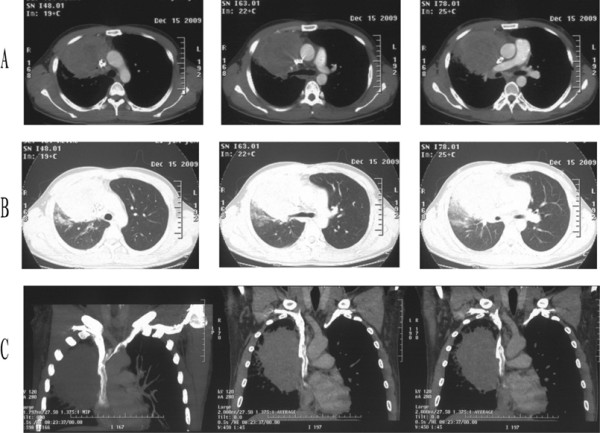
Enhanced chest CT scan before operation. (A) In the mediastinal window, the CT scan revealed the tumor encroaching on the superior vena cava (right panel), surrounding the right upper lobe bronchus (middle panel), and invading the right pulmonary artery (left panel). (B) In the lung window. (C) In the mediastinal window, coronal.
